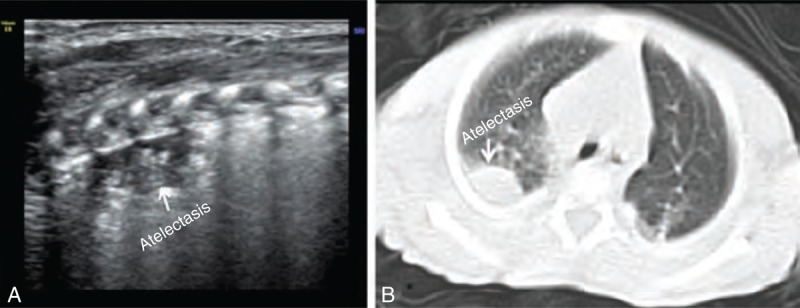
Pulmonary occult atelectasis. GA 26^+2^ weeks, birth weight 900 g, hospitalized because of RDS. This patient required a ventilator for 58 days and oxygen supplementation for 100 days and was diagnosed with BPD clinically. A lung ultrasound showed (A) consolidation and an air bronchogram within 2 intercostal ranges, (B) findings that were confirmed as atelectasis by chest CT scan. This occult atelectasis was the reason for this baby's LTOD. BPD = bronchopulmonary dysplasia, GA = gestational age, LTOD = long-term oxygen dependence, RDS = respiratory distress syndrome.
